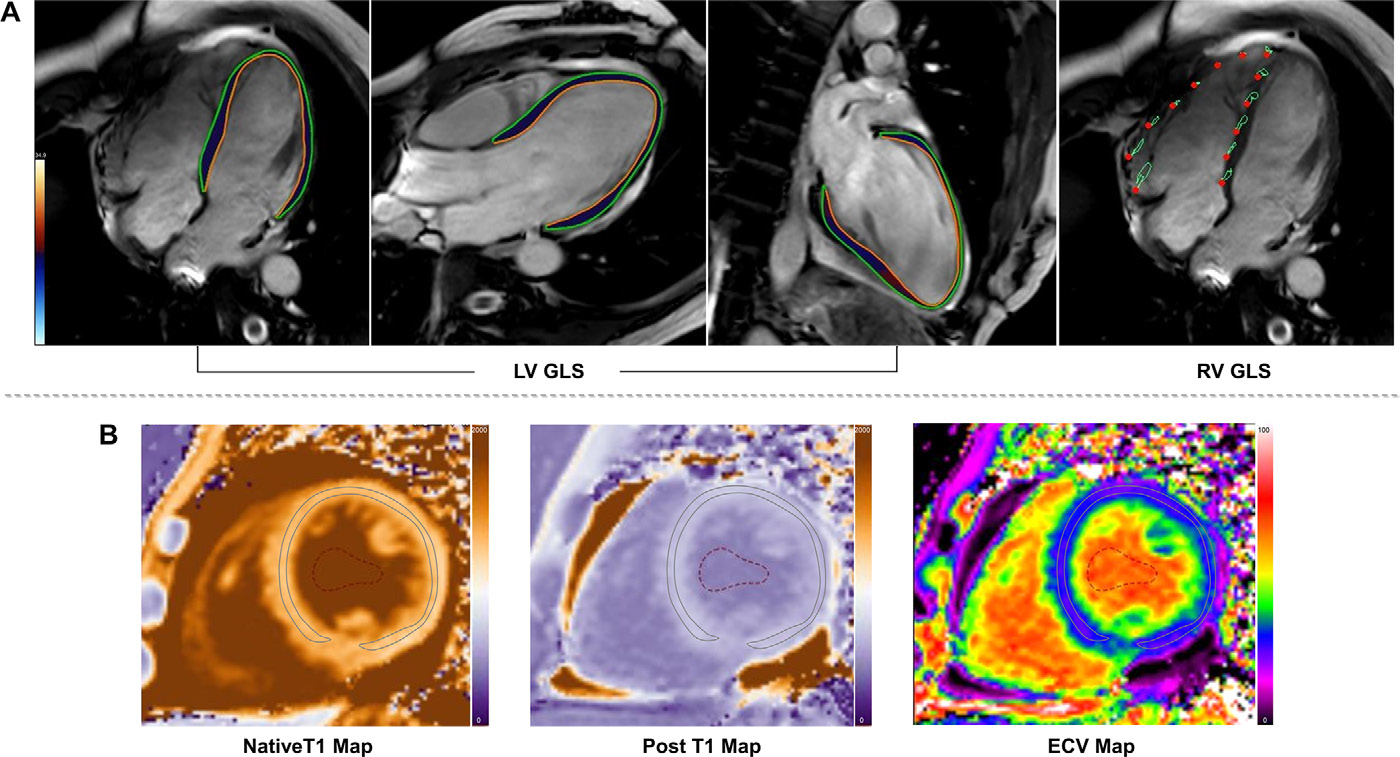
Images of cardiac MRI biventricular global longitudinal peak strain (GLS)
